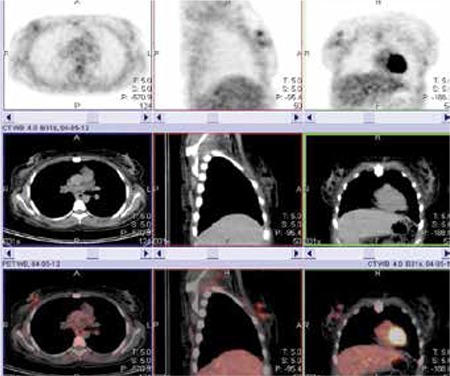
The patient was scanned by an integrated PET/CT camera (one hour after the administration of 465 MBq FDG), which consists of a 6-slice CT gantry integrated on a LSO based full ring PET scanner (Siemens Biograph 6, IL, Chicago, USA). MIP PET, CT and fusion PET/CT images were obtained. There were 2 focal lesions with moderate metabolic activity (SUV max of 3.6) in the upper inner quadrant of the right breast.
